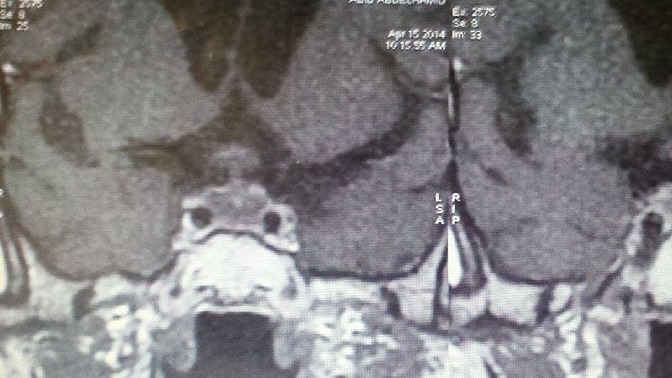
Magnetic resonance imaging: an inhomogeneous pituitary hypertrophy with convexity of the sellar diaphragm
